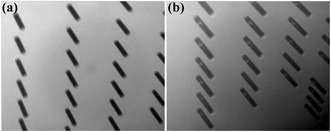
X-ray images of TSVs after Cu electroplating. a TSVs without Cu filling failure. b TSVs with Cu filling failure caused by the Cu seed layer loss due to the sponge-like defects at 30 μm × 150 μm TSV [45]
